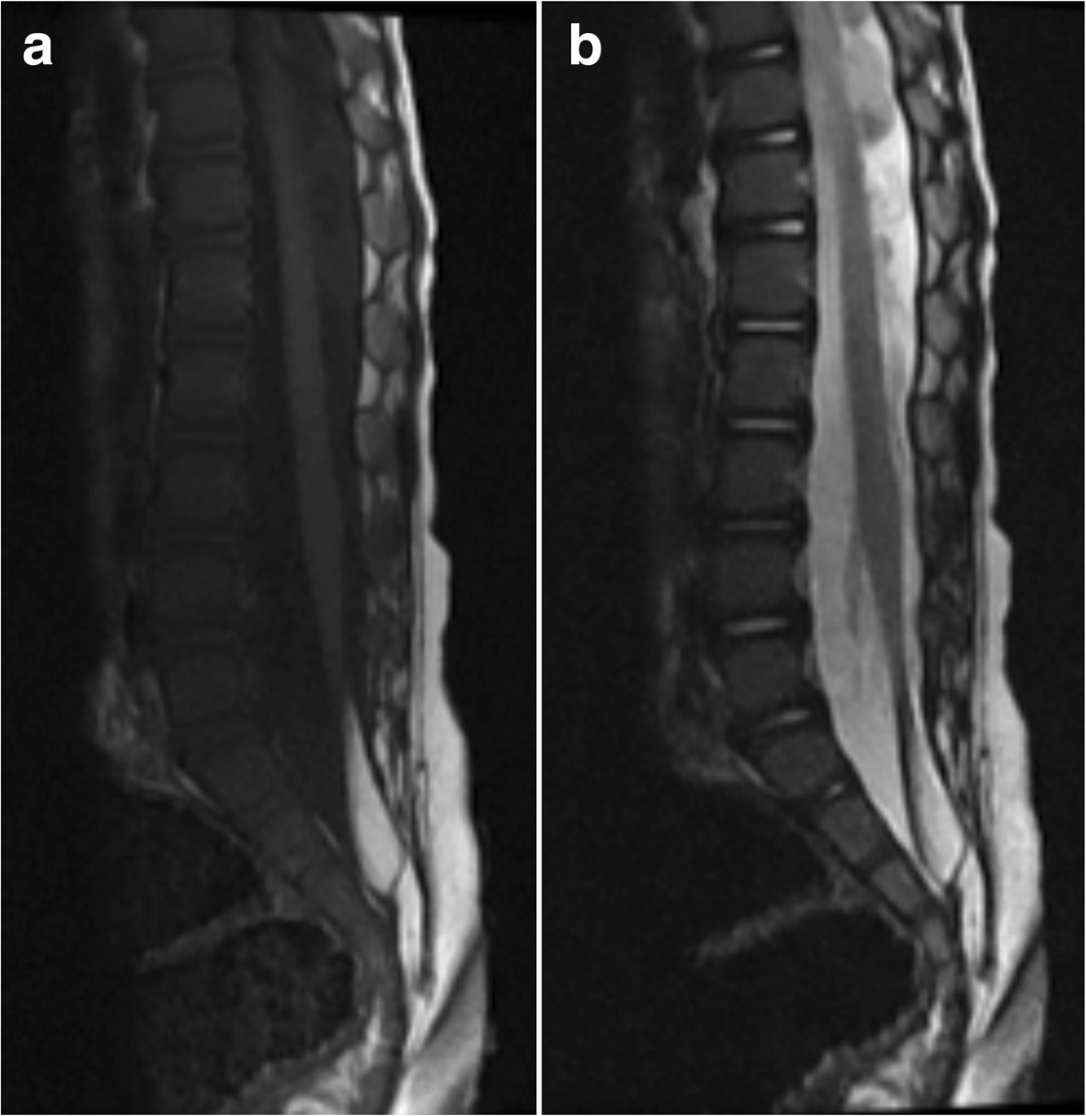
a. T1 weighted MR images demonstrating low conus, fatty filum, and sacral intradural lipoma b. T2 weighted MR images demonstrating low conus, fatty filum, and sacral intradural lipoma
